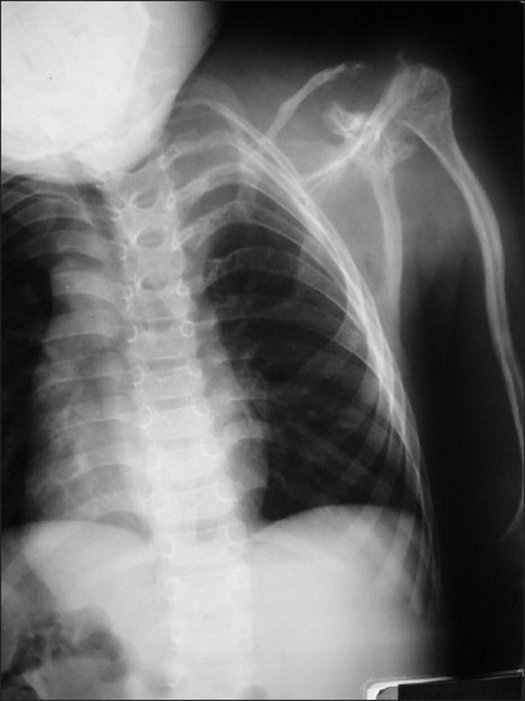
The radiograph shows dysplastic left clavicle, humerus and the first four ribs
